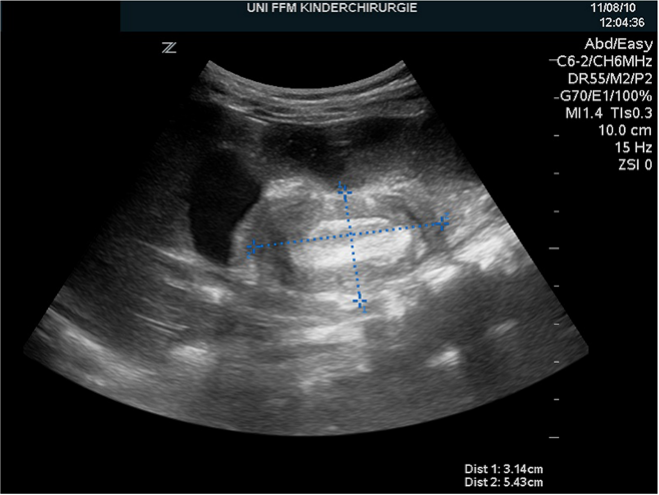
Ultrasonography of the right upper abdominal quadrant revealed the typical ultrasonographic finding of “pseudo-kidney”.
